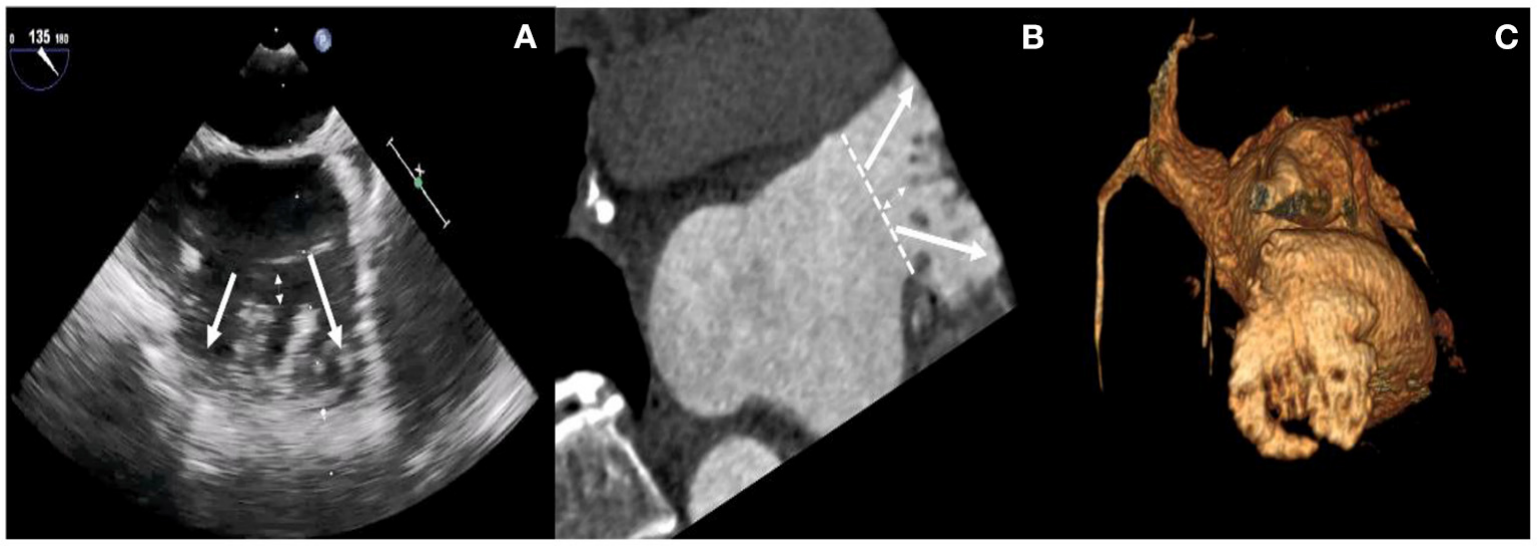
Higher degrees of 2D TEE and CCTA images. TEE views at 120° (A) reveal the presence of two main lobes with short common distances to the common ostium, a great diameter of ostium, and pectinate muscles that separated the LAA into multiple lobes (white arrows). Multiplanar reconstruction (B) and volume-rendering computed tomography (CT) image (C) provide an overview of the anatomy of LAA lobes and pectinate muscles.
